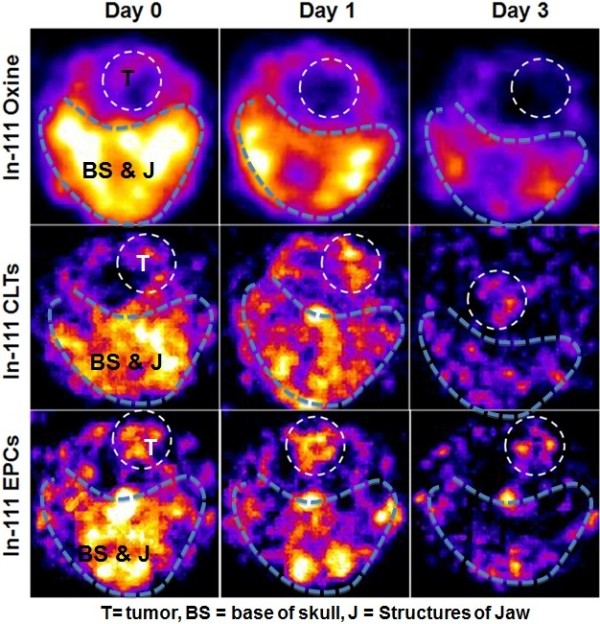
SPECT images of biodistribution of labeled cells in brain region. In-111 oxine alone did not show any activity around the tumor area. Accumulation of In-111 labeled CTLs in tumor area was seen within three hours and the activity further increased on 24 hour scans. In-111 labeled EPCs showed high activity around the tumor area in 3 hours scan, which was significantly higher compared to CTLs. 24 hours scan showed further increase in the radioactivity. Note: The increased accumulation of cells after 24 hours of injection. Window levels of signals are kept identical for all day for respective tumors.
